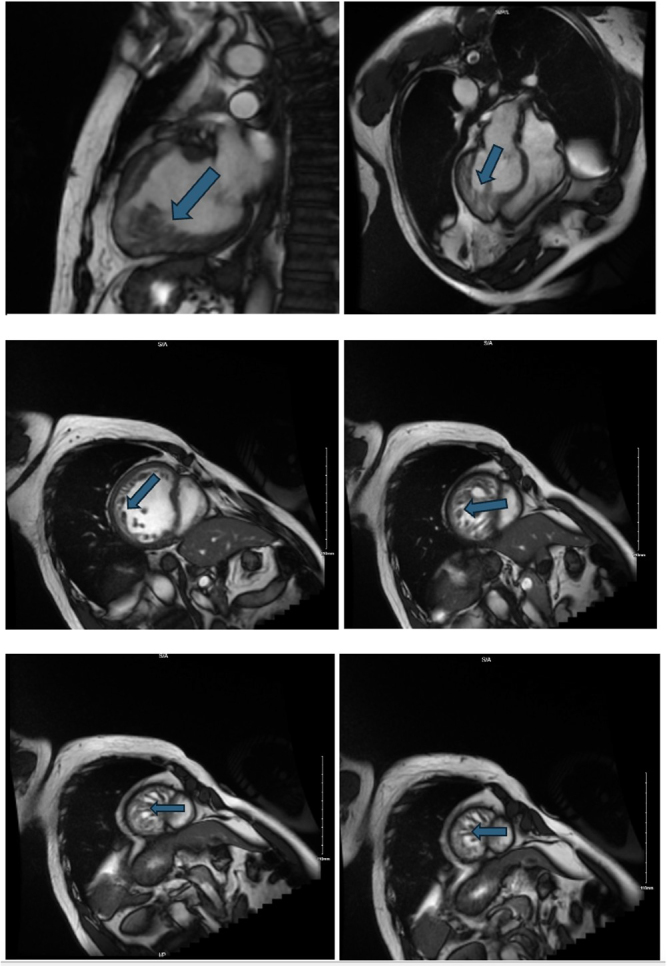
Cardiac MRI. Image (A) Hypertrabeculation in anatomical LV (sagittal localizer image). Images (B–F) Hypertrabeculation is more apparent in the mid-apical part (short axis bSSFP cine images).
